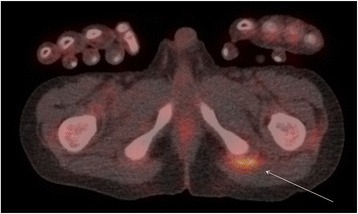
Detailed image of fludeoxyglucose positron emission tomography-computed tomography scan showing increased fludeoxyglucose uptake in the left ischiopubic tendon attachments (*arrow*)
